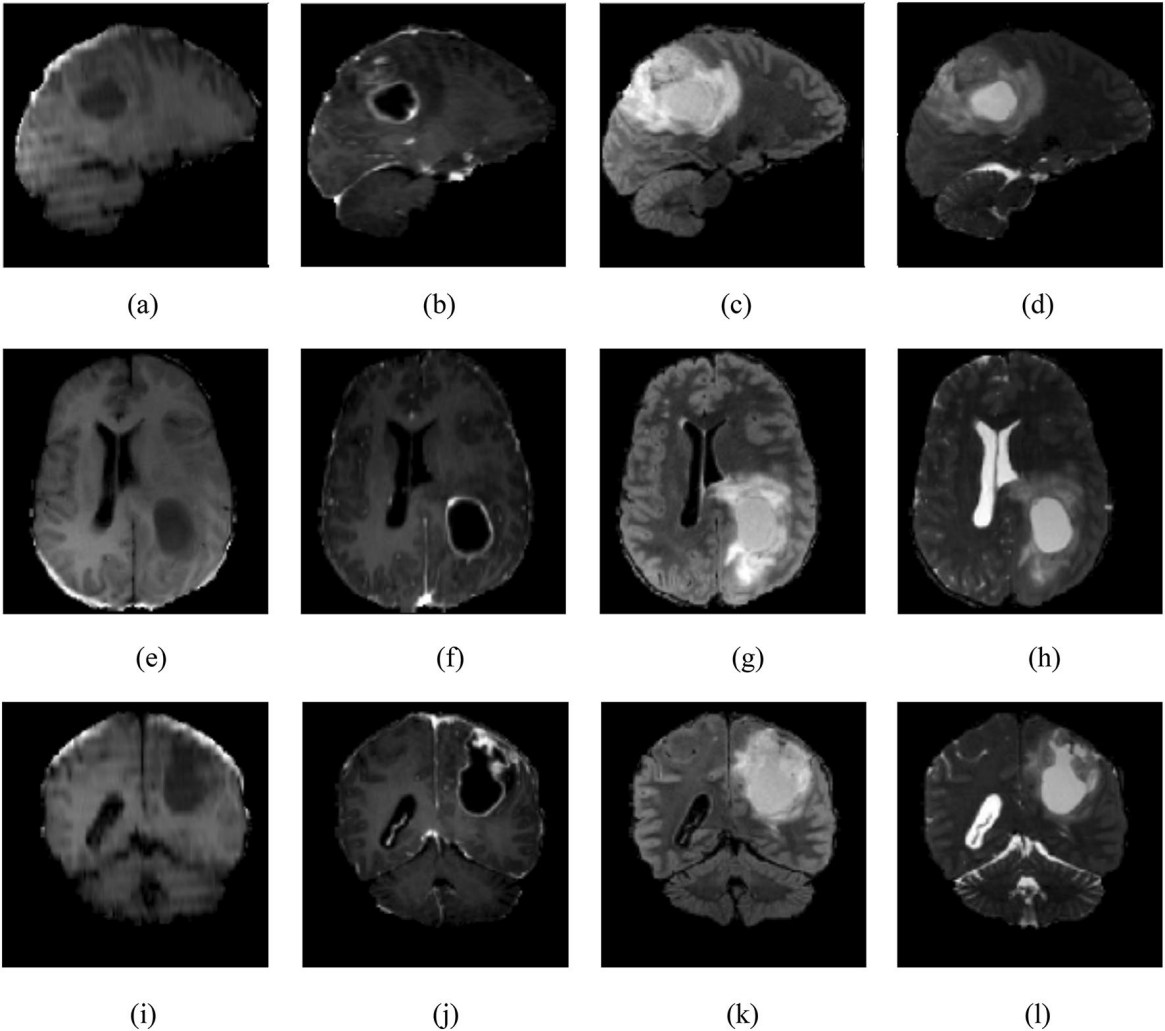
BraTS-2021 mpMRI scans from top to bottom: sagittal, axial and coronal view: first, second and third columns show (a,e,i) T1w, (b,f,j) T1wCE, (c,g,k) FLAIR, and (d,h,l) T2w images respectively.
